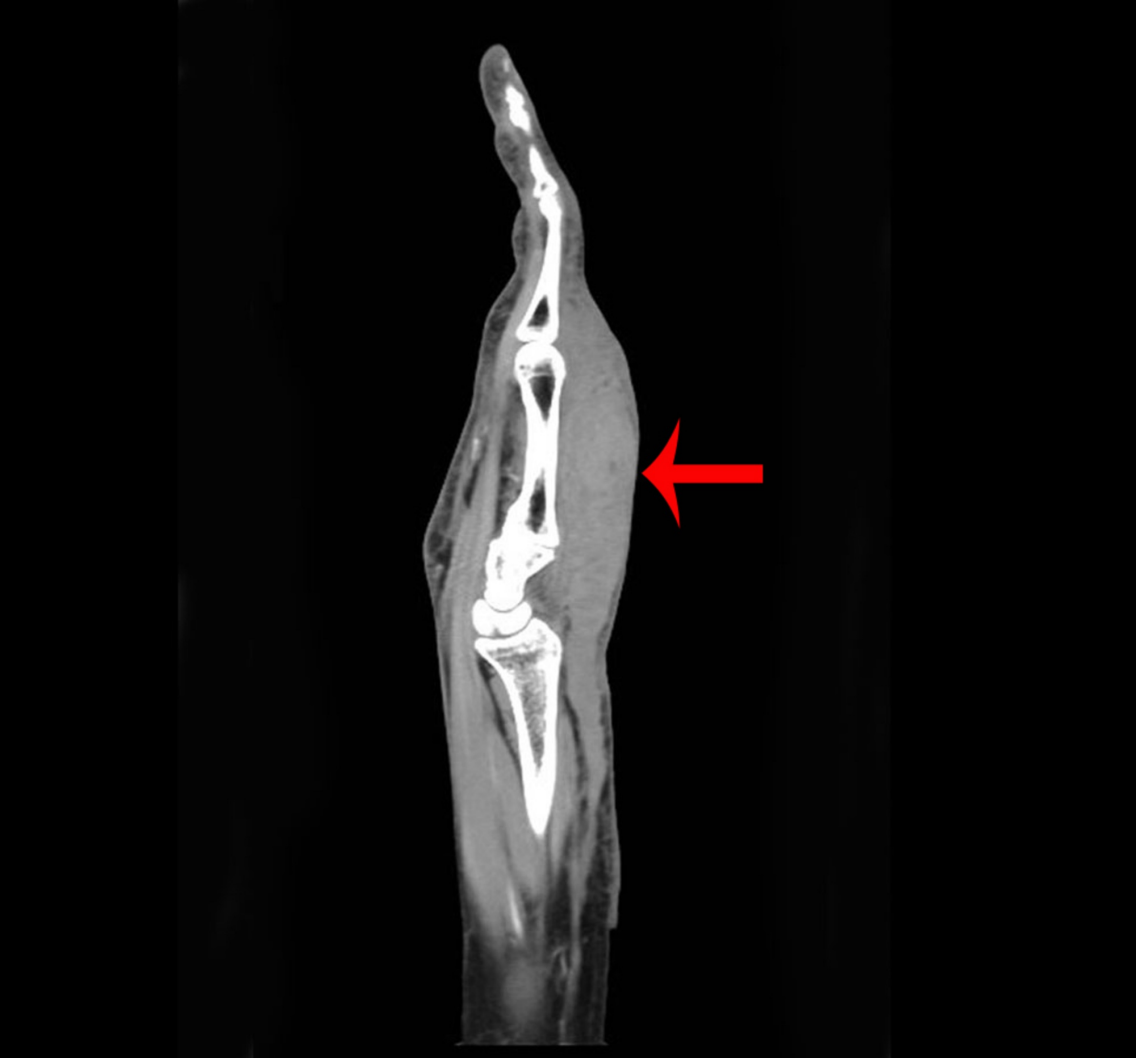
CT scan of hand (coronal view) CT: computed tomography
